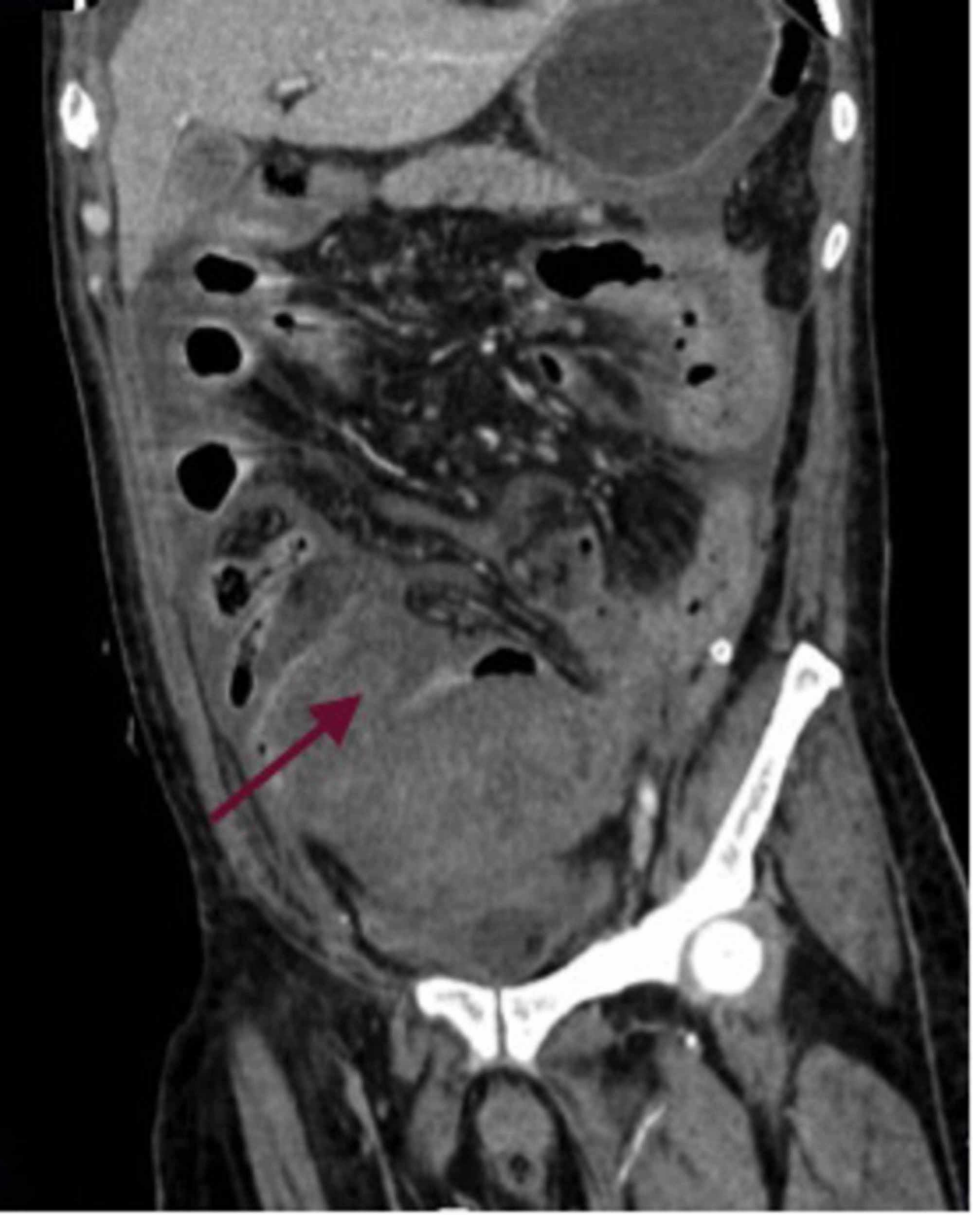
Coronal view contrast-enhanced computed tomography (CECT) of the abdomen showing pancolonic oedema (arrow)
